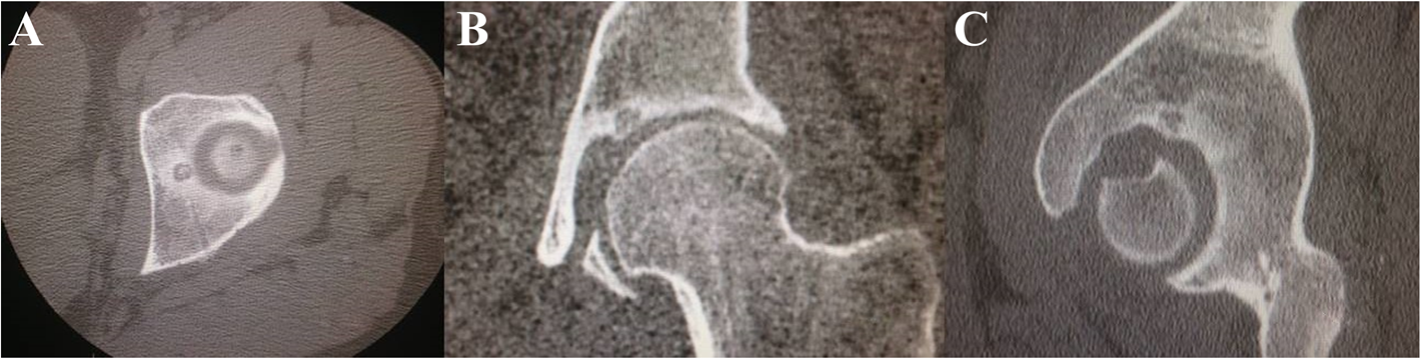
Axial (a), coronal (b) and sagittal (c) views of a computed tomography scan showing a round nidus of osteoid osteoma at the superomedial aspect of the left acetabulum, lying immediately superior and posterior to the fovea in a 31 year-old male with left hip pain
